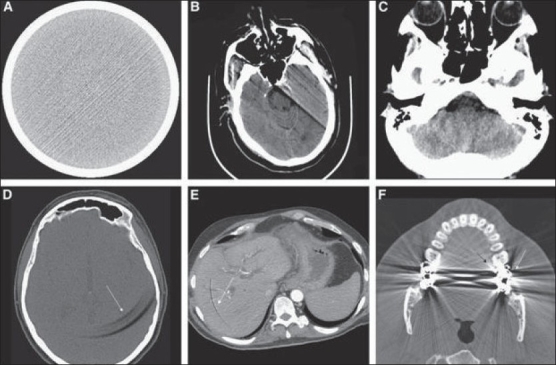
Examples of CT Artifacts: (A) Streak (B) Motion (C) Beam-hardening (D-E) Ring (F) Bloom [4]
